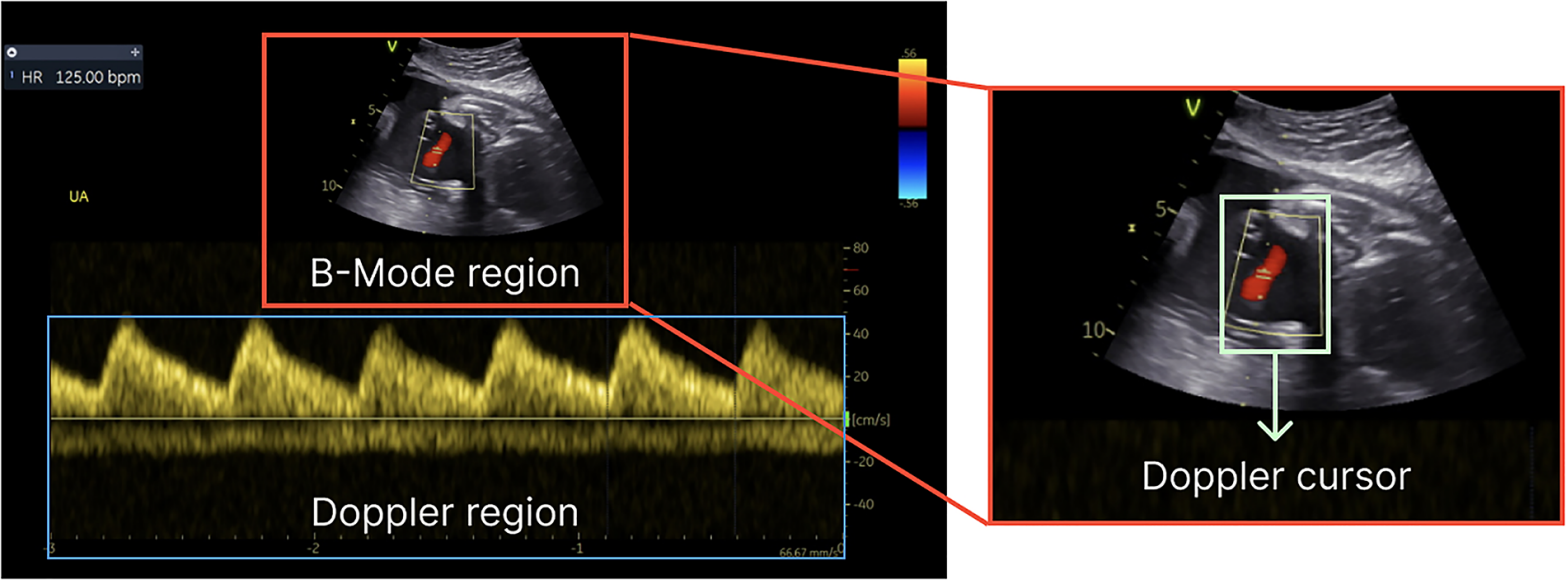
Components of a Doppler image—B-mode for structural details, the Doppler region for blood flow information and Doppler cursor on top of the B-mode region.
